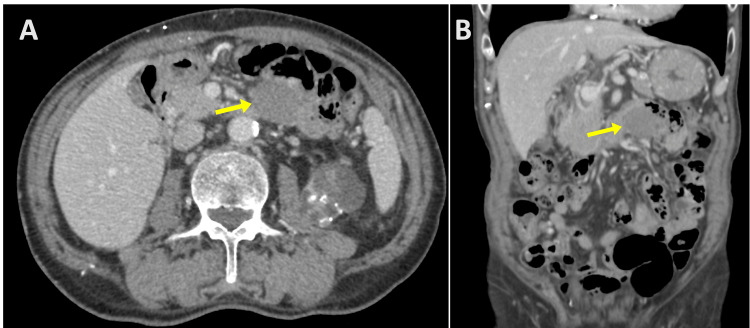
Contrast-enhanced CT images of the proximal jejunal tumor. (A) Axial and (B) coronal views reveal a well-circumscribed, homogenous mass measuring 36 × 30 × 35 mm located in the proximal jejunum. The mass exhibited mild and uniform enhancement, with no signs of invasion into adjacent organs.
